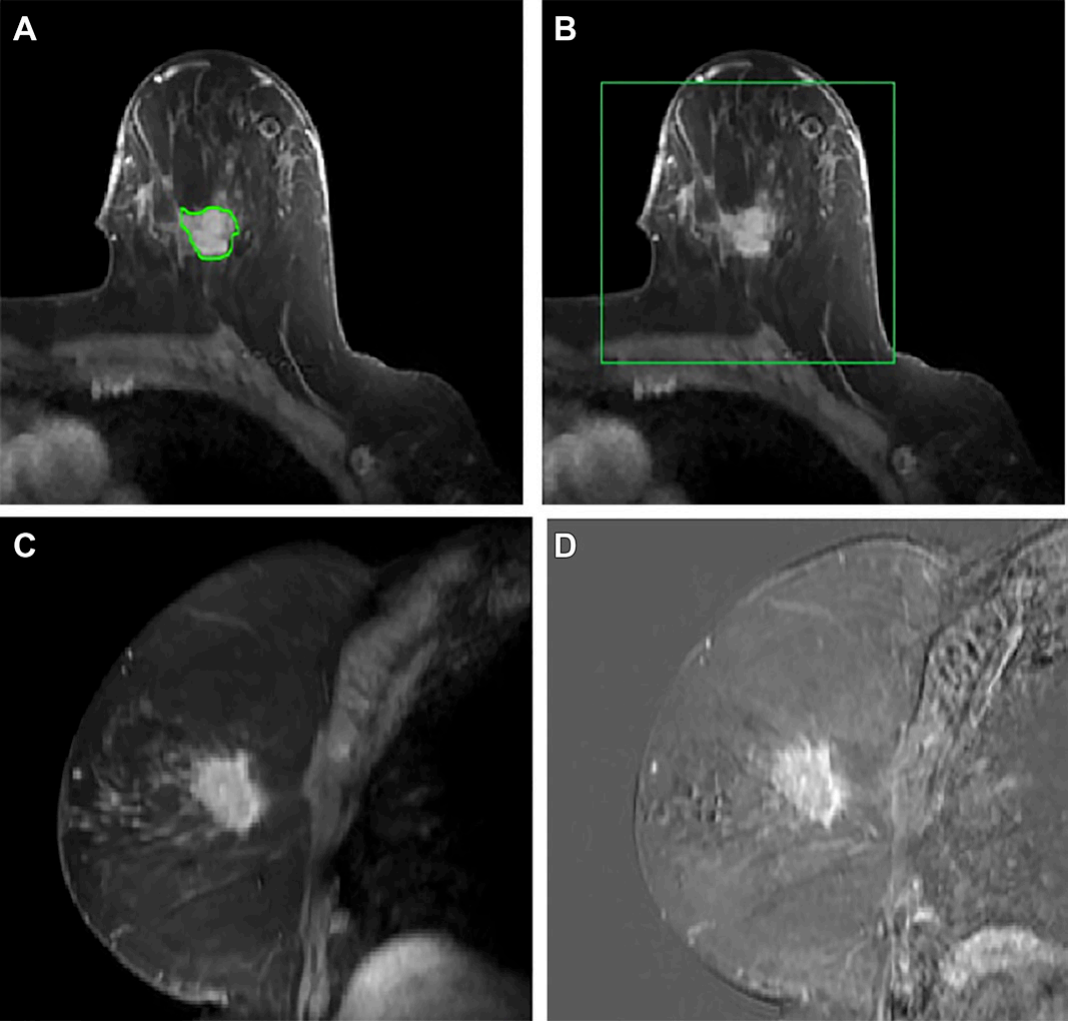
Preprocessing a volumetric dynamic contrast-enhanced MR image.
